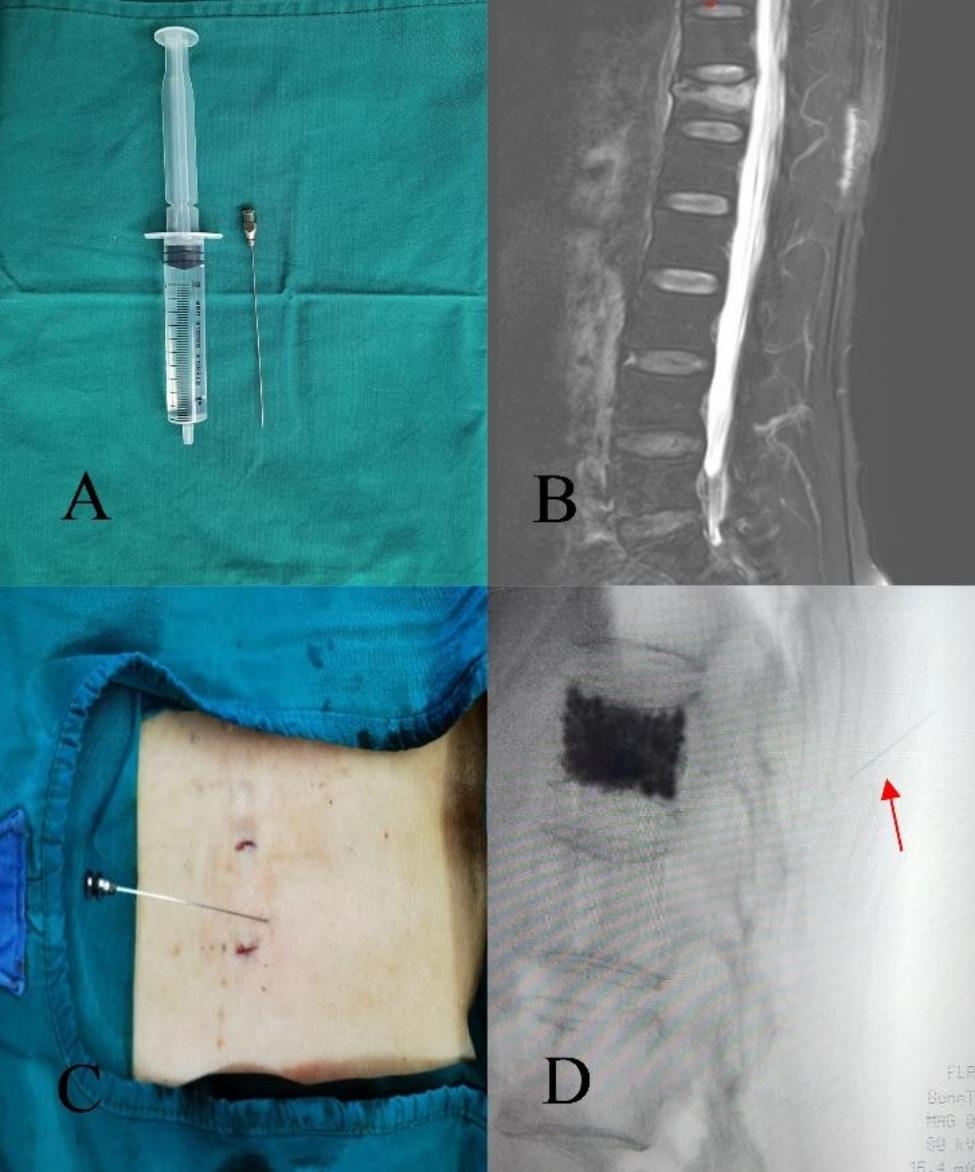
A 22-gauge needle and a 10 ml syringe (A) were applied to inject the cocktail; MRI of T2-weighted fat suppression image (B) showed T12 vertebral compression fracture with TFI; The needle guided by the C-arm X-ray machine (C, D) was used to locate and inject the cocktail in the area of TFI after PVP (The red arrow represented the needle)
